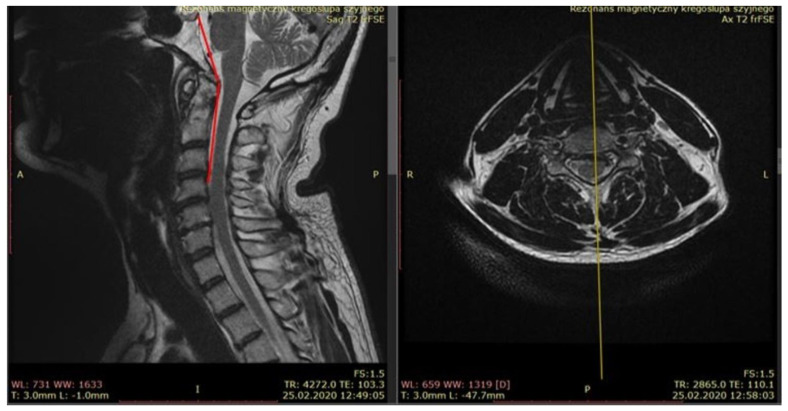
CXA in magnetic resonance imaging (MRI).
